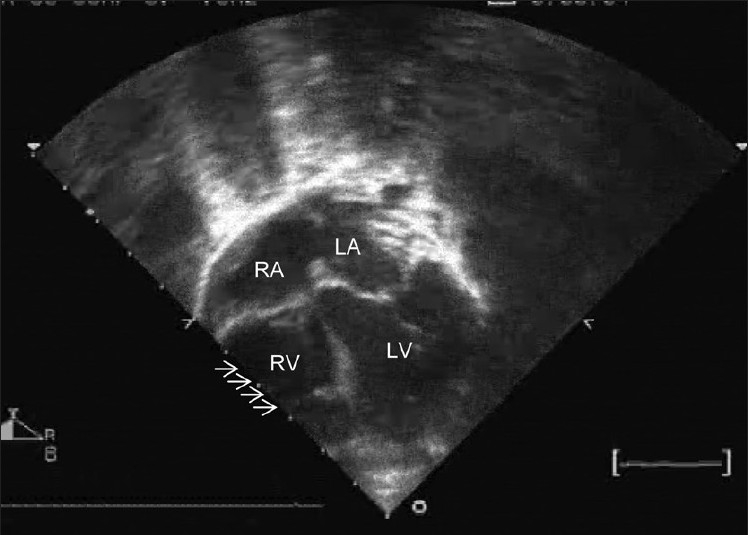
Echocardiogram showing a large ASD with hypoplastic right ventricle (marked with arrows)
